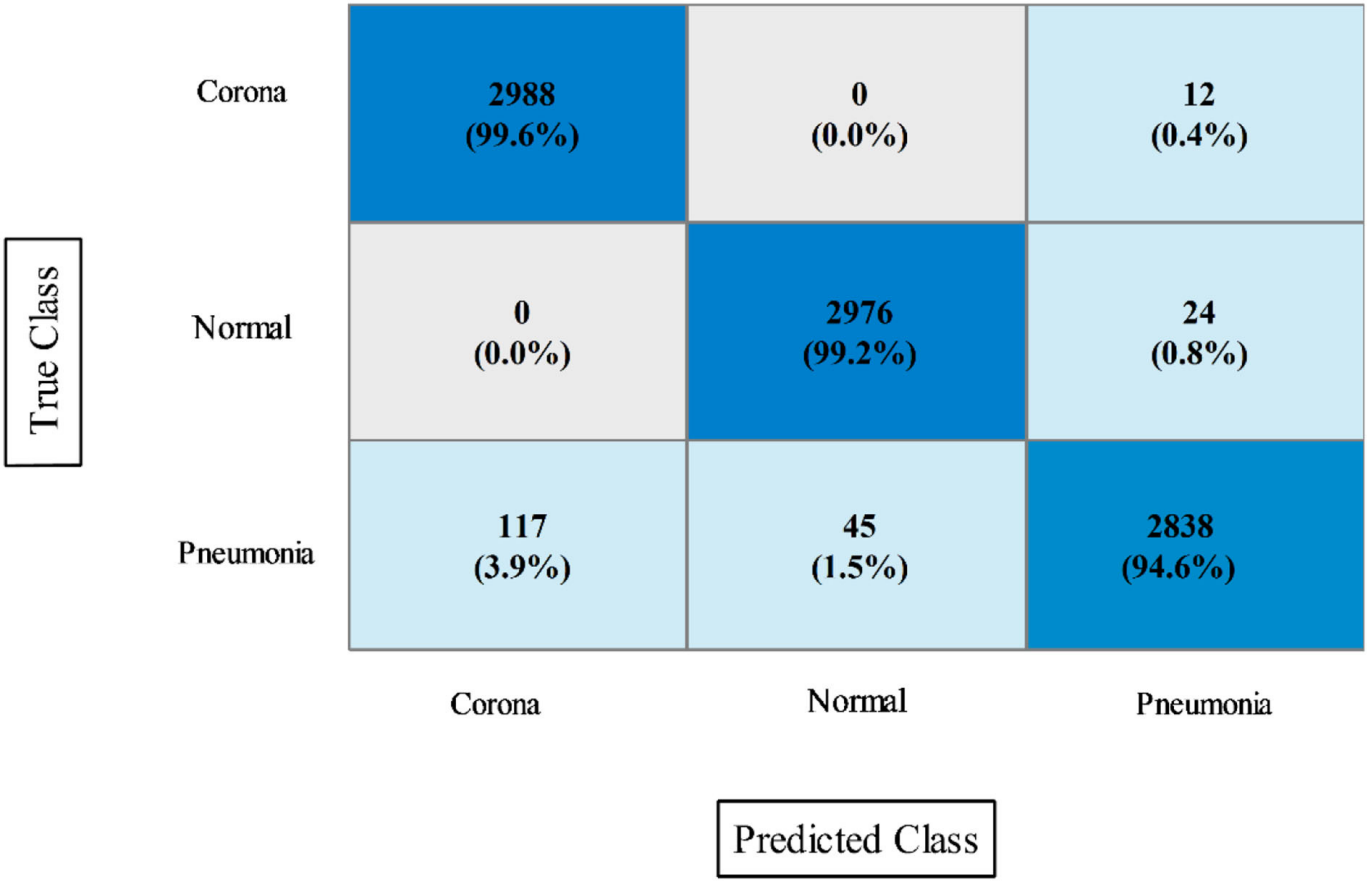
Confusion matrix of CSVM after proposed features fusion for COVID-GAN and COVID-Net mini chest X-ray dataset.
